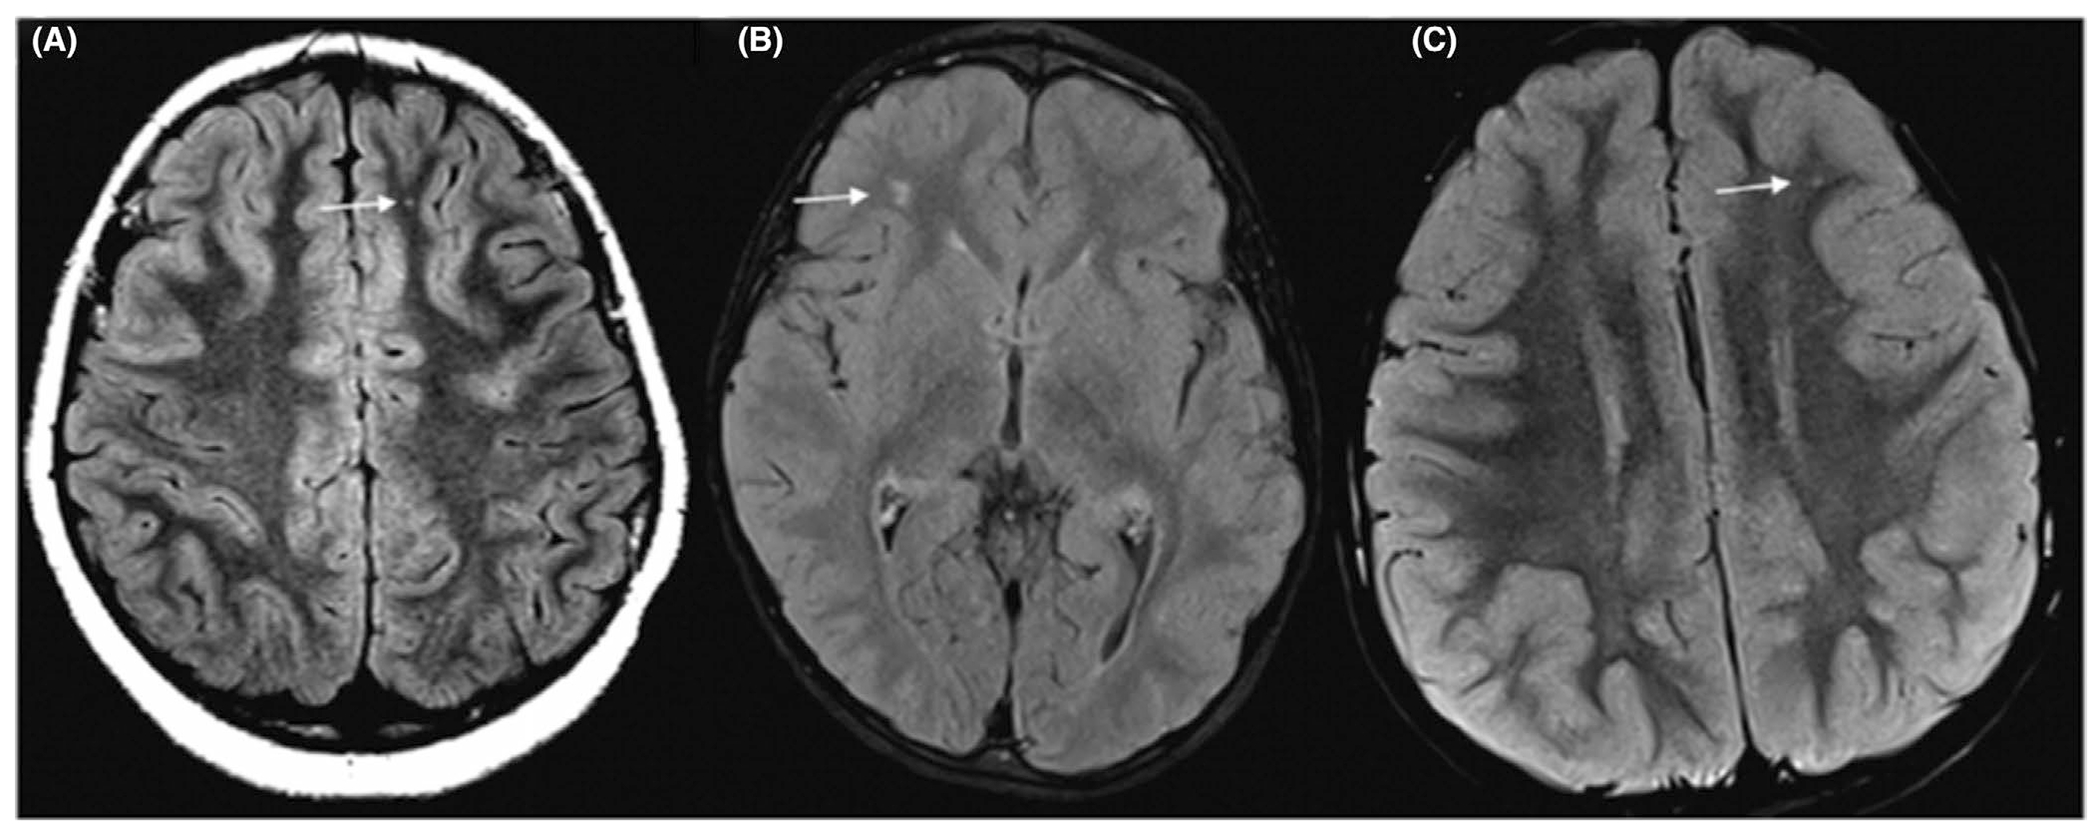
Brain MRI images using axial T2-weighted fluid-attenutated inversion recovery sequences are shown for 3 patients with CVS and migraine headaches who show evidence of hyperintensities in the subcortical white matter (arrows). From reference 27.
